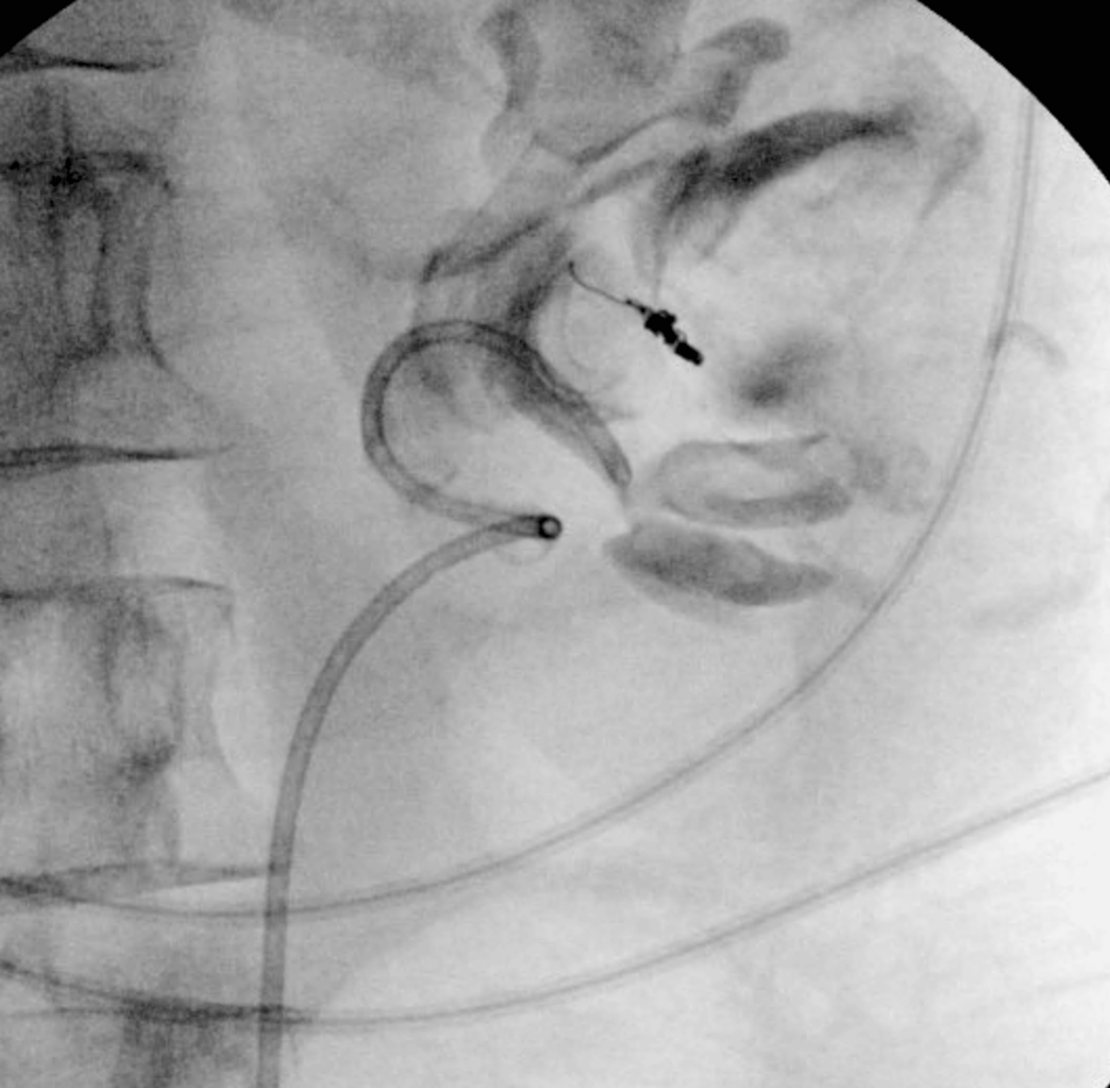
X-ray showing the left ureteric stent in situ
